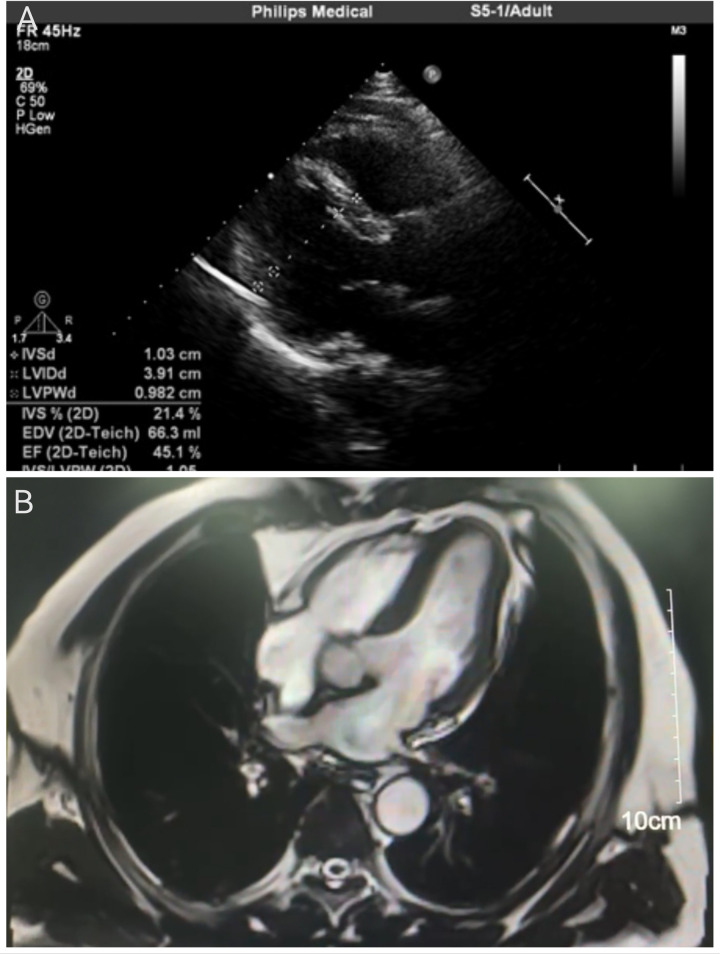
Cardiac imaging assessments. (A) Transthoracic echocardiogram (parasternal long-axis view) indicating a structurally normal heart. Quantitative measurements are displayed, including a left ventricular ejection fraction (LVEF) of 45.1%. (B) Cardiac magnetic resonance image (four-chamber view) with late gadolinium enhancement. The homogeneous myocardial signal and the scale bar (10 cm) confirm the absence of edema, fibrosis, or structural abnormalities.
